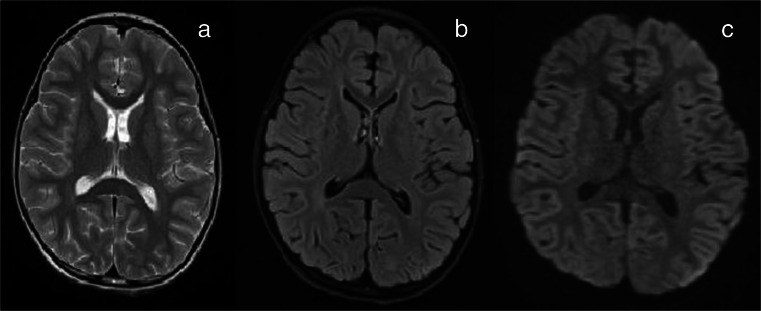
Magnetic resonance imaging (*MRI*) obtained on the tenth day of illness shows complete disappearance of the splenial white matter lesions (MRI Axial T2W (a), FLAIR (b), DWI (c))
